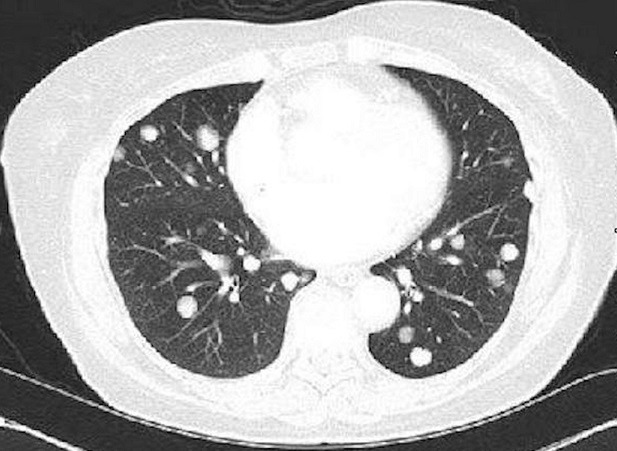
CT chest showing bilateral pulmonary metastases
